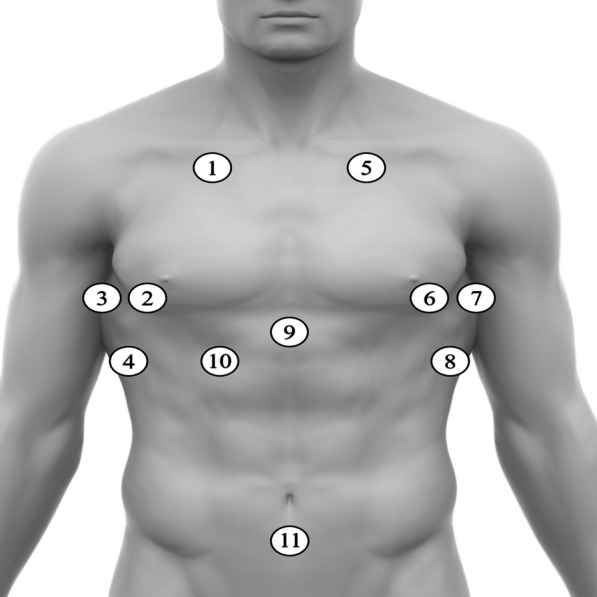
Sites examined by point-of-care ultrasound for evidence of plasma leakage in patients with suspected dengue
